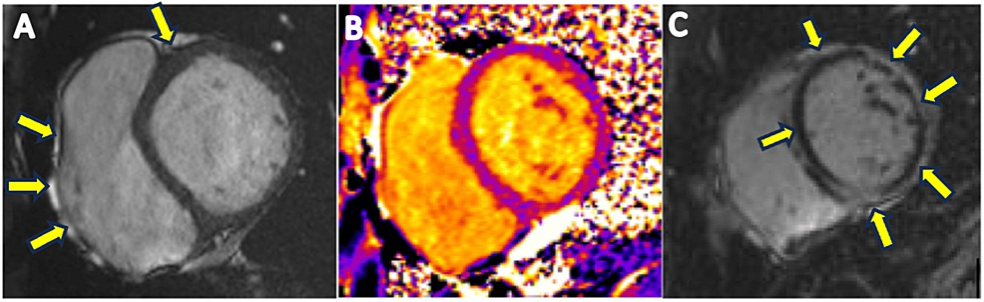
Short axes images of cardiovascular magnetic resonance images showing abnormal delayed enhancement (arrows).
